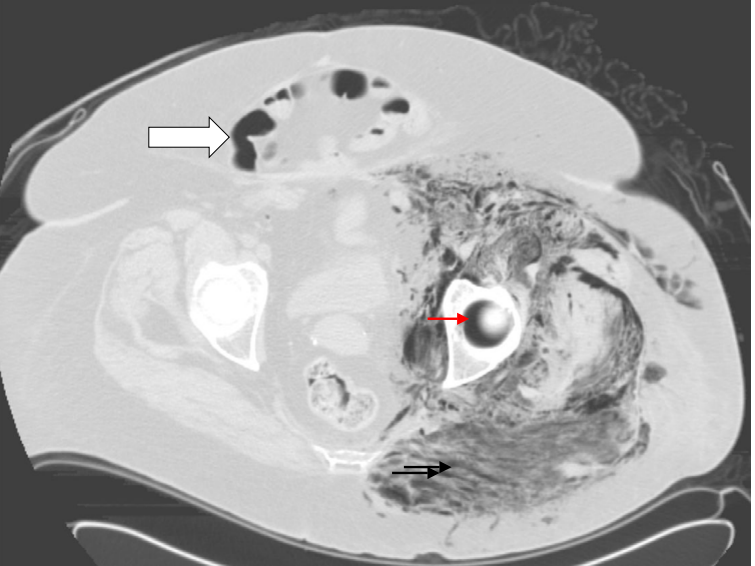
CT scan of the pelvis confirming the extensive gaseous formation tracking along the fascial planes characteristic of myonecrosis *(black arrows)*. The formed gas also extends into the acetabular gap, giving the crescentic appearance *(red arrow)*. Note the marked adiposity in the form of anterior fat-apron with a ventral wall hernia *(large arrow)*.
